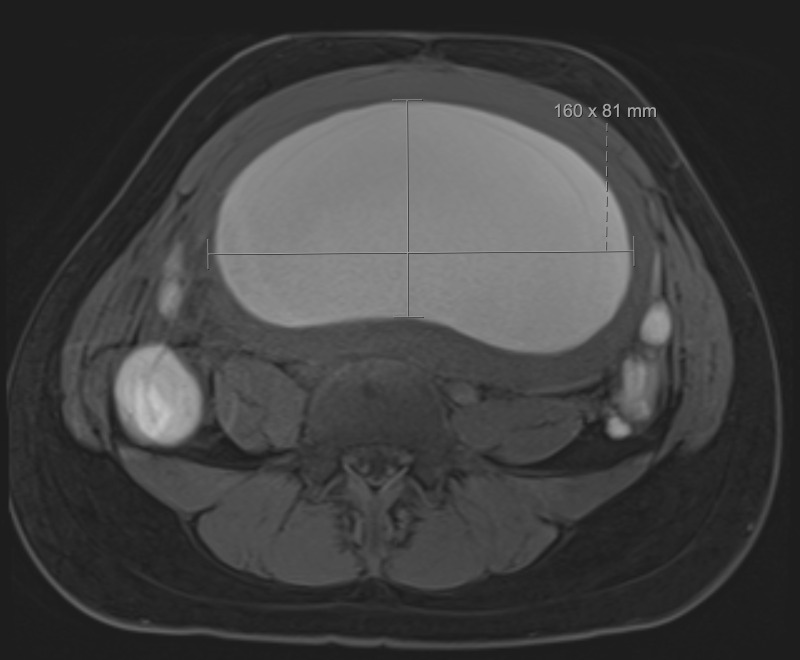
T1 Weighted Axial MRI Image.
